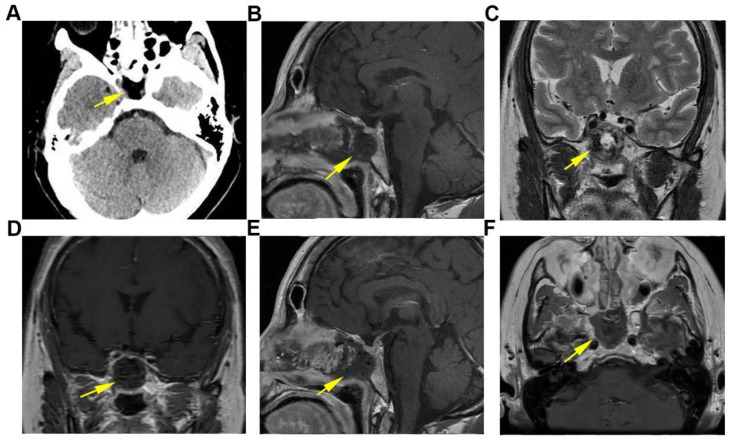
Postoperative imaging follow-up. (A) Axial non-contrast cranial CT image. (B–E) Cranial MR images: (B) Sagittal T1-weighted image. (C) Coronal T2-weighted image. (D) Coronal T1-weighted contrast-enhanced image. (E) Sagittal T1-weighted contrast-enhanced image. (F) Axial T1-weighted contrast-enhanced image. These images collectively indicate the complete resection of the lesion located in the sphenoid sinus region, with a well-healed surgical site and no discernible evidence of residual tumor.
